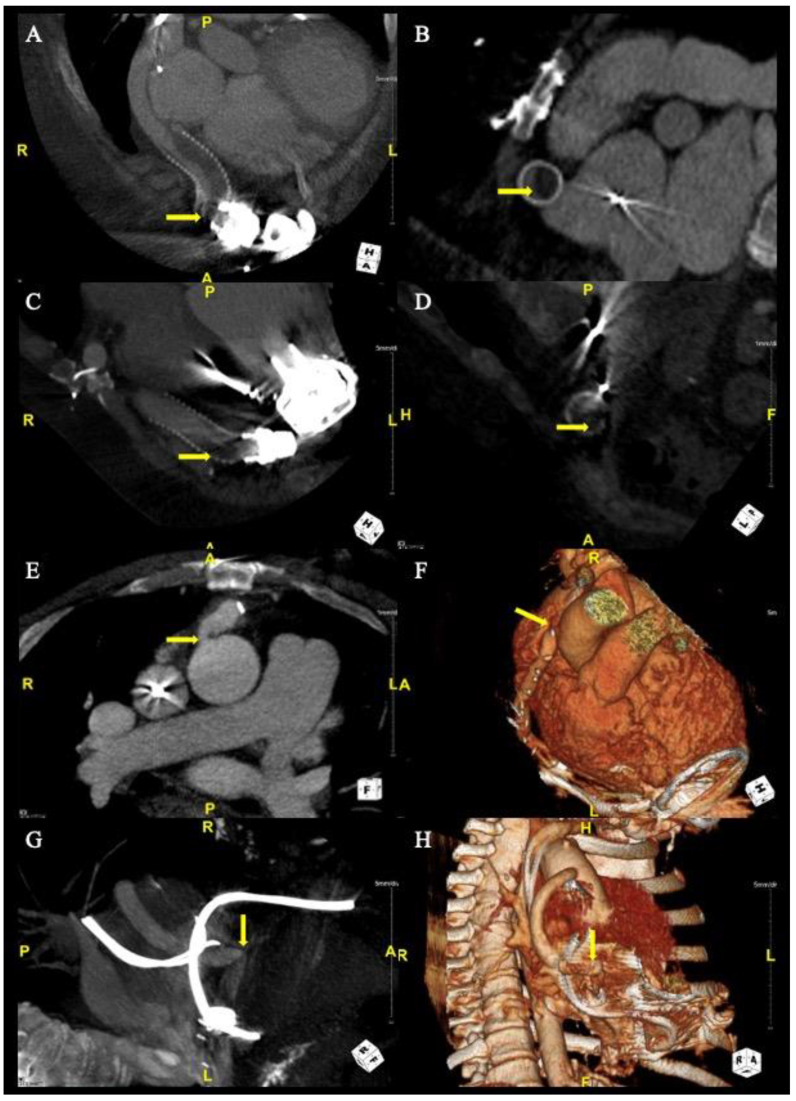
Computed Tomography Angiography of Obstructions. For case 1, CTA (Panel A) demonstrates stenosis with bend relief disconnect and CTA (Panel B) demonstrates a partially occlusive thrombus. For case 5, CTAs (Panel C,D) demonstrate partially occlusive thrombus. For case 8, CTAs (Panel E,F) demonstrate ascending aorta anastomotic stenosis. For case 11, CTAs (Panel G,H) demonstrate outflow cannula kink.
